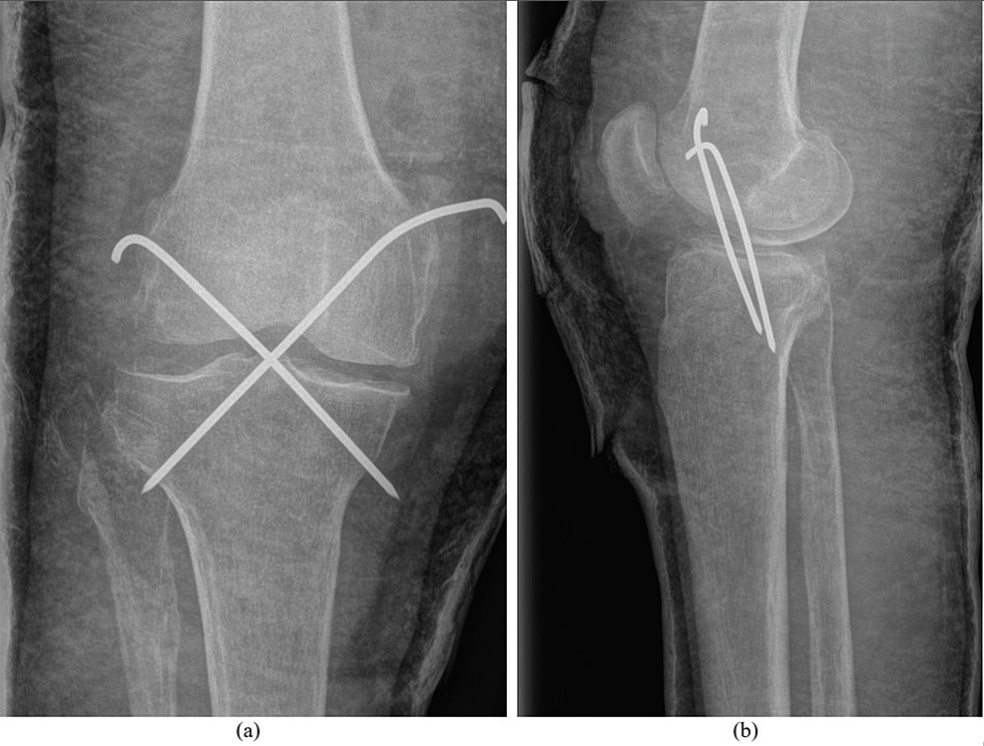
Temporary stabilization using K-wires and cast immobilization of the right knee, anteroposterior and lateral radiographs.
